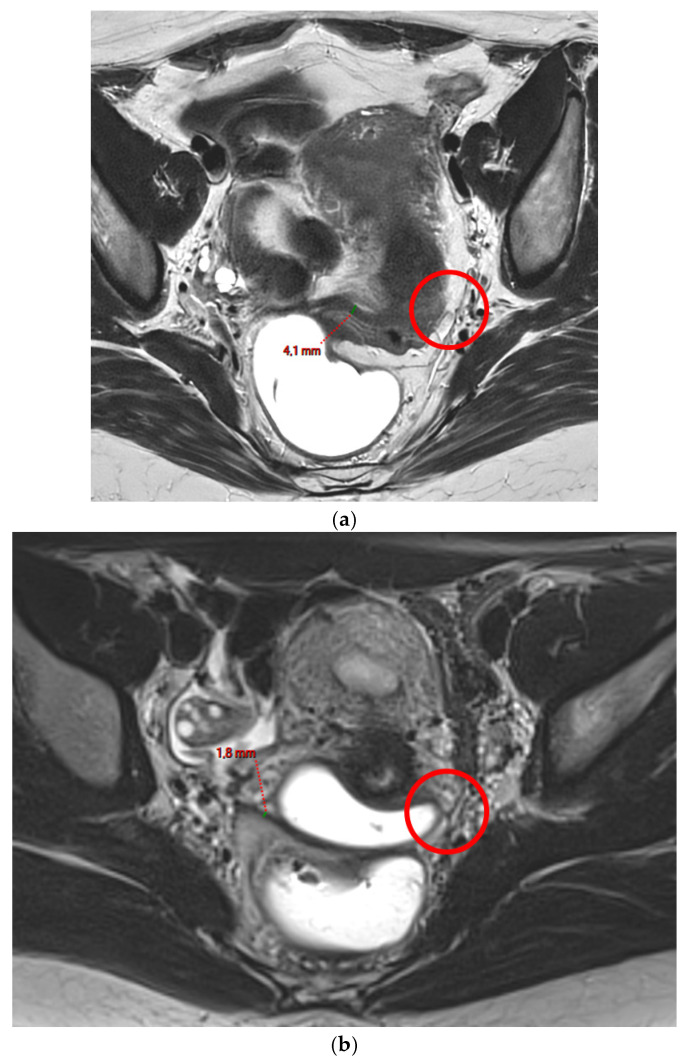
Pelvic MRI scans of two patients with non-visible left USLs (HTD type 1). (a) Axial T2WI shows a visible and measurable right USL but a non-visible left USL (*red circle*). (b) Axial T2WI shows a visible and measurable right USL but a non-visible left USL (*red circle*).
